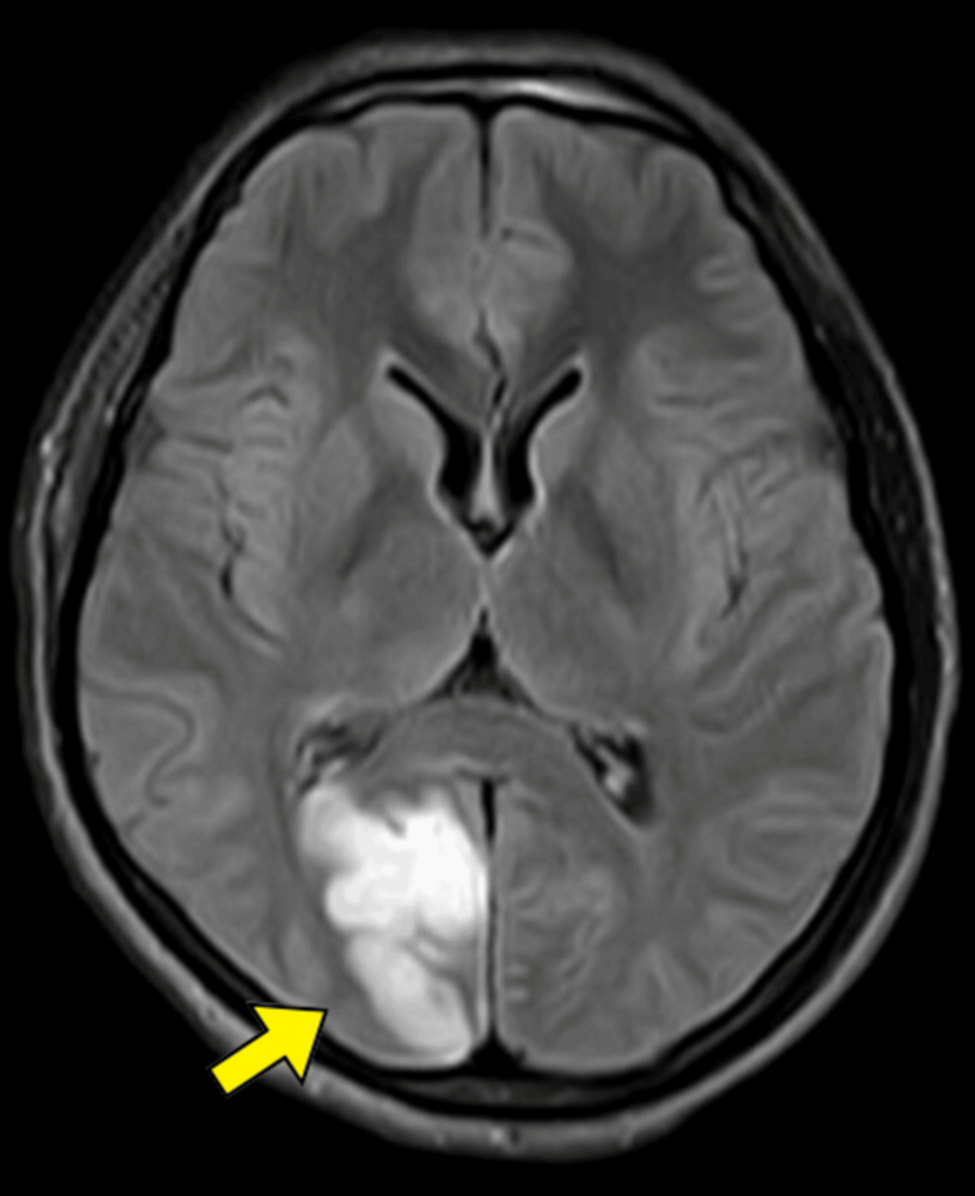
Magnetic resonance imaging (MRI) of the brain showing hyperintensity right occipital lobe (yellow arrow).
